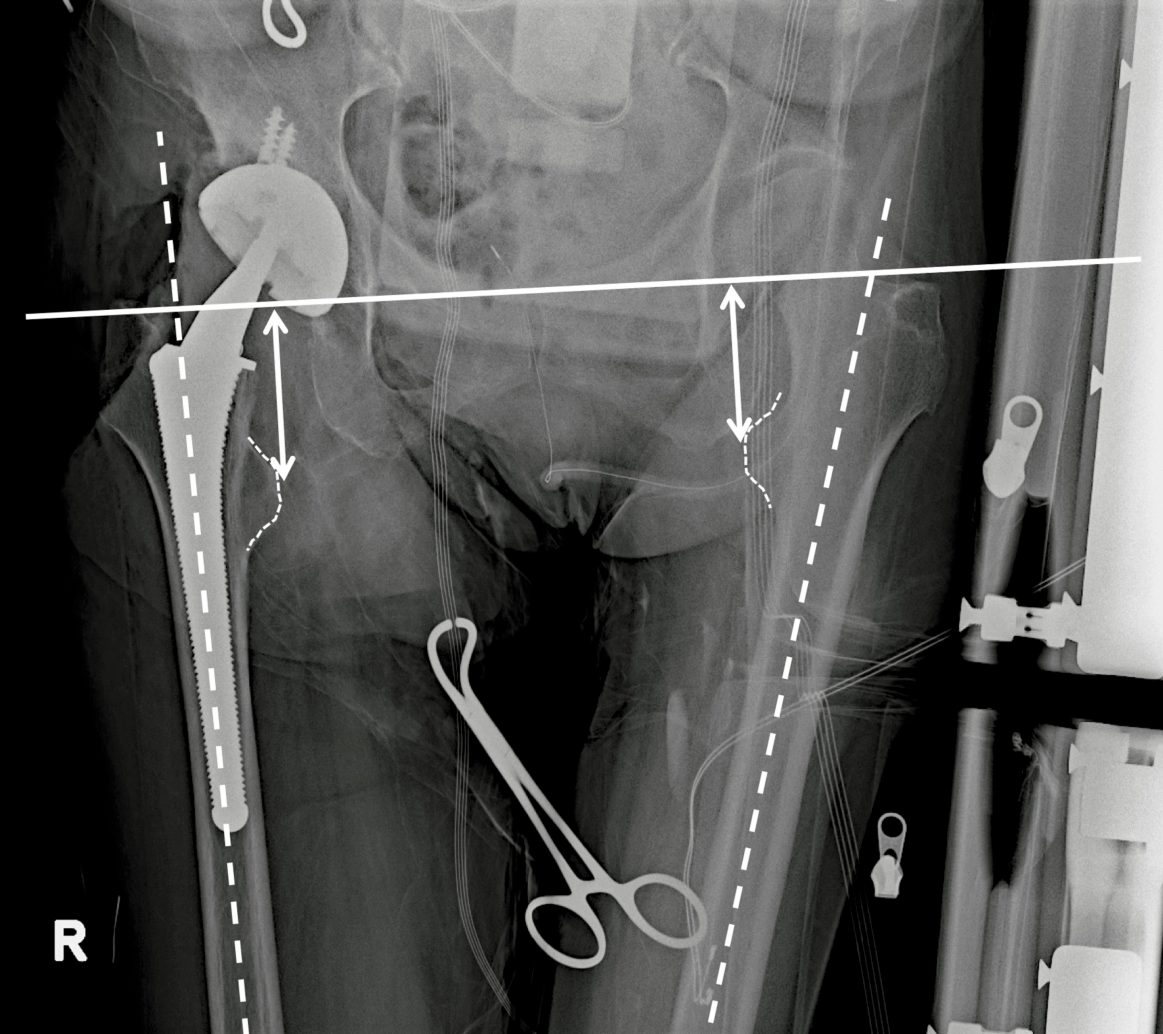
Perioperative radiograph Measurement of leg length on a perioperative radiograph. The vertical distance (arrows) is measured from the trans-teardrop line (the solid line) to the most prominent point of the lesser trochanter. The broken line indicates the femoral anatomical axis.
